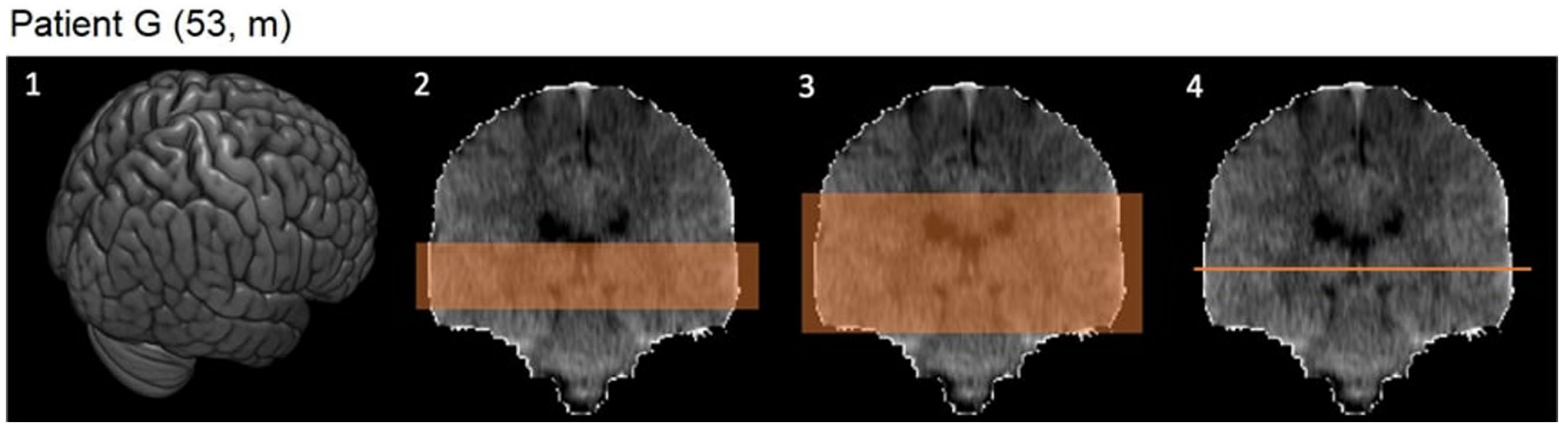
CT images in 3D and coronal reconstruction. 1: 3D image of the whole brain. 2: Image stack of 50 slices including the basal ganglia. 3: Image stack of 100 slices. 4: One slice at the level of the anterior commissure.
